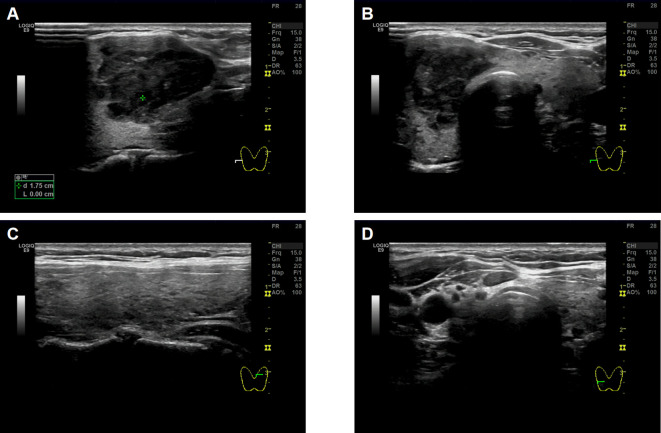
Preoperative thyroid and cervical ultrasound findings. (A) A nodule in the right thyroid lobe with ill-defined margins and irregular shape, measuring approximately 29 mm in diameter. (B) The thyroid gland exhibits heterogeneous hypoechogenicity with coarsened echotexture. (C) A small nodule in the left thyroid lobe with well-defined margins and regular shape, measuring approximately 3.5 mm in diameter, categorized as C-TIRADS 3. (D) A hypoechoic lymph node was noted at level VI of the right neck.
